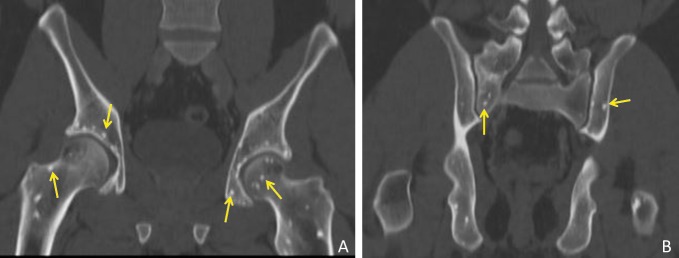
Coronal Reformatted Computed Tomography (CT) of the Pelvis. Small non-aggressive appearing sclerotic foci (yellow arrows) are visualized involving the periarticular (A) right and left hip and (B) sacroiliac joints.
